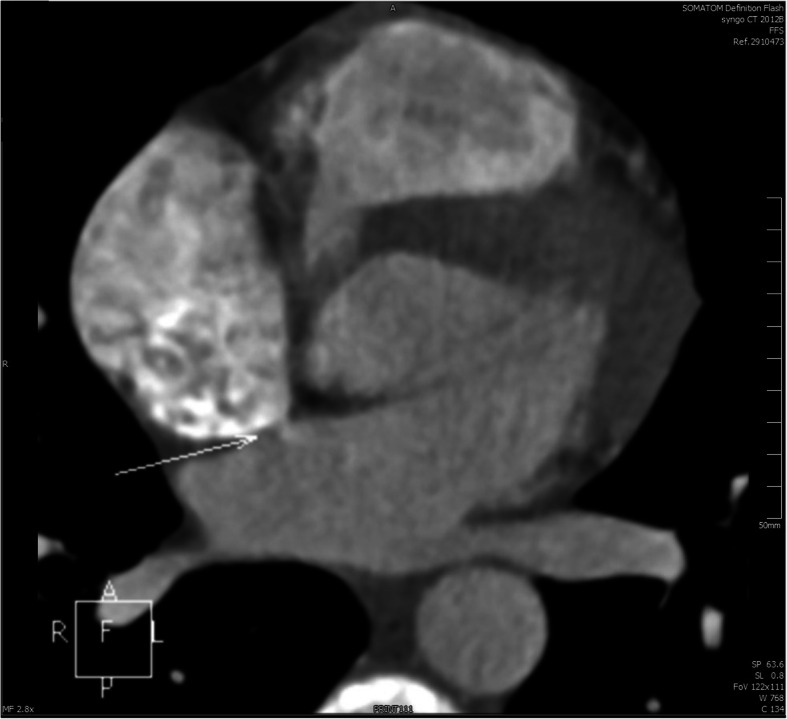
Before transcatheter closure, the cardiac CT showed small ASD
